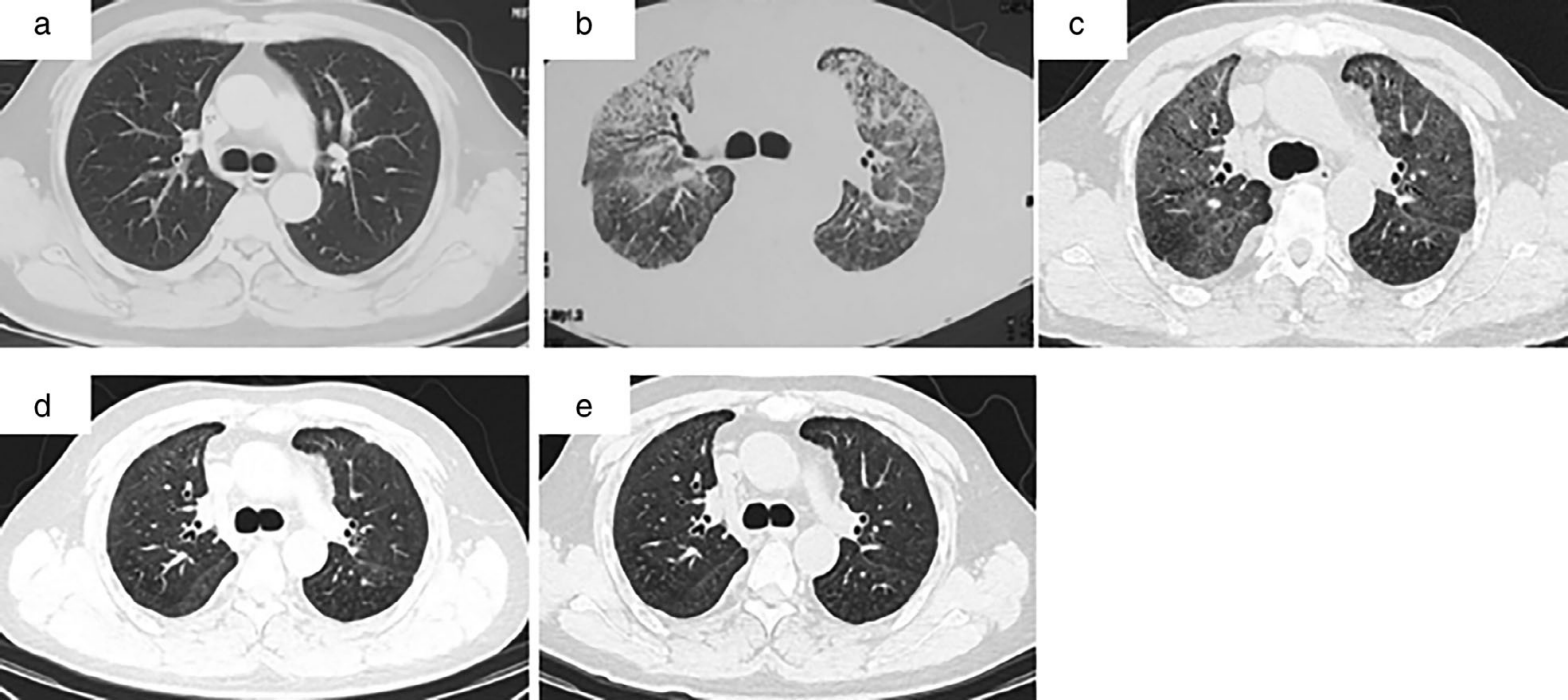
Series chest computed tomography (CT) imaging during the treatment. (a) There was no significant abnormal opacities visible on chest CT in February 2018. (b) The repeat high‐resolution CT (HRCT) scan of his chest in June 2018 showed diffuse bilateral GGOs without obvious lymphadenopathy. (c–e) The pulmonary GGOs gradually disappeared on the repeat chest HRCT scans six weeks, six months and 12 months after prednisone administration.
